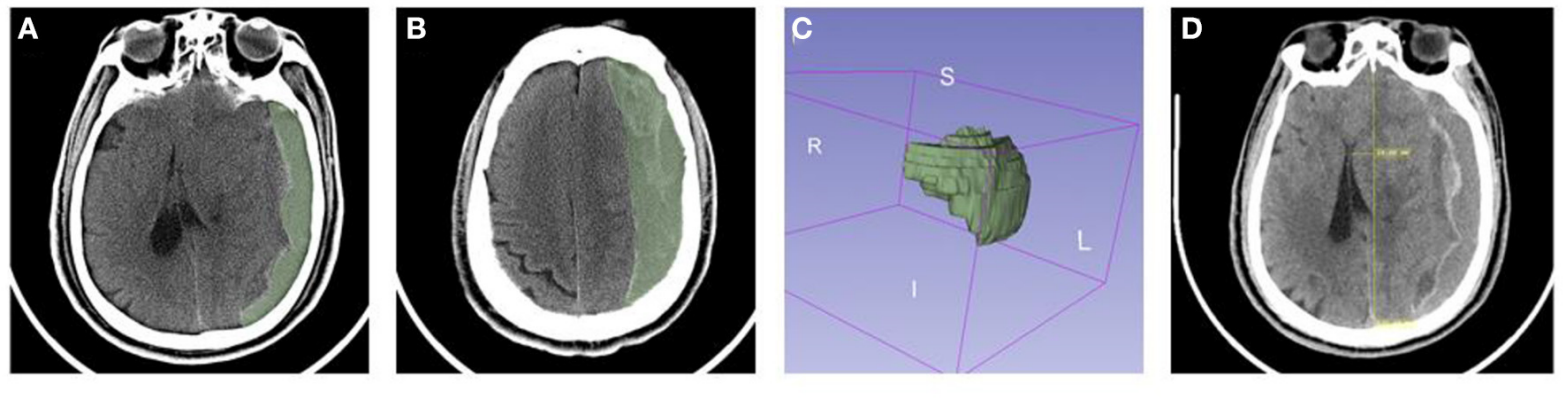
The CT images of CSDH with mixed density were analyzed with 3D slicer. (A–C) Calculation of the volume of hematoma. (D) Measurement of mid line shift.
